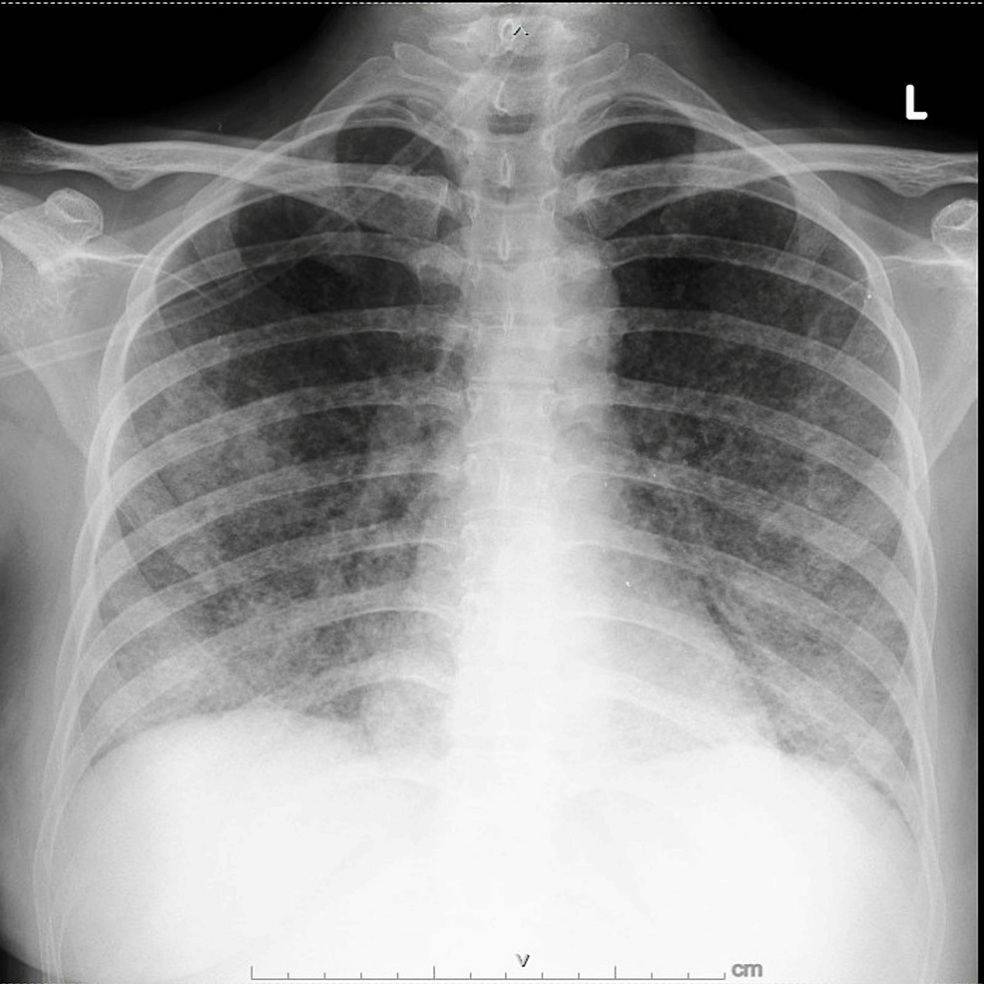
Chest X-ray in the referral hospital
